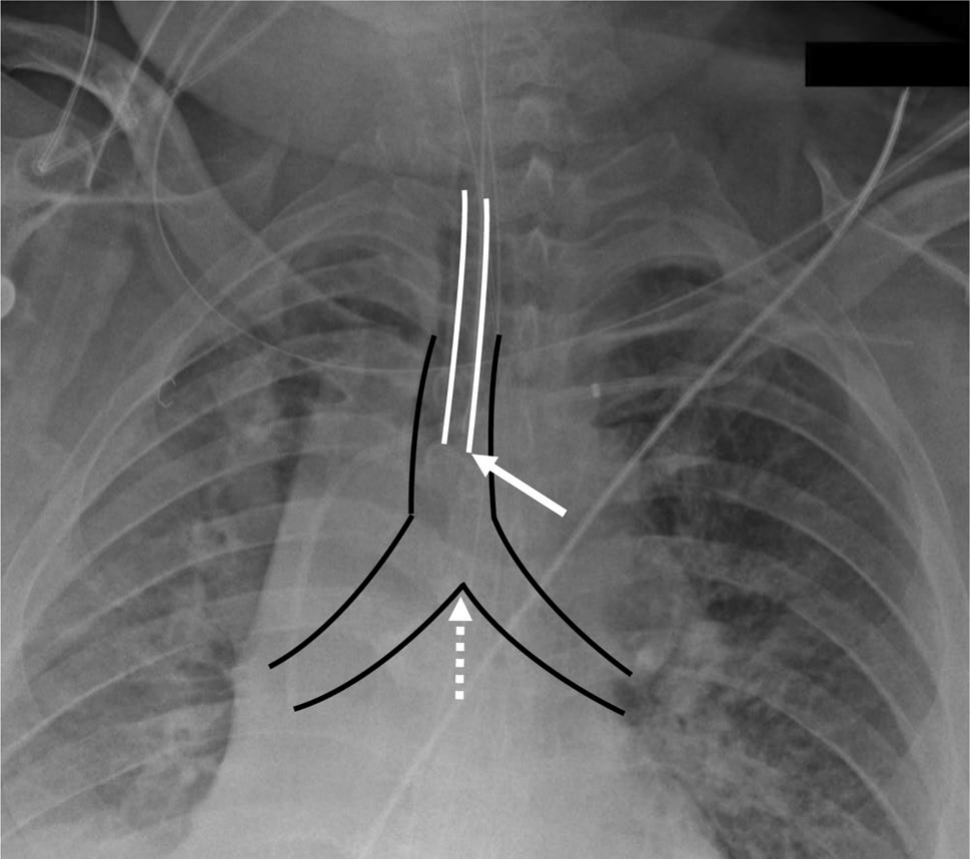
Frontal chest radiograph Single frontal radiograph demonstrating position of an endotracheal tube (ETT; solid white outline) relative to the trachea and proximal right and left bronchi (solid black outline). Optimal positioning is commonly evaluated by characterizing the distal ETT tip (solid white arrow) relative to the carina (dotted white arrow)
